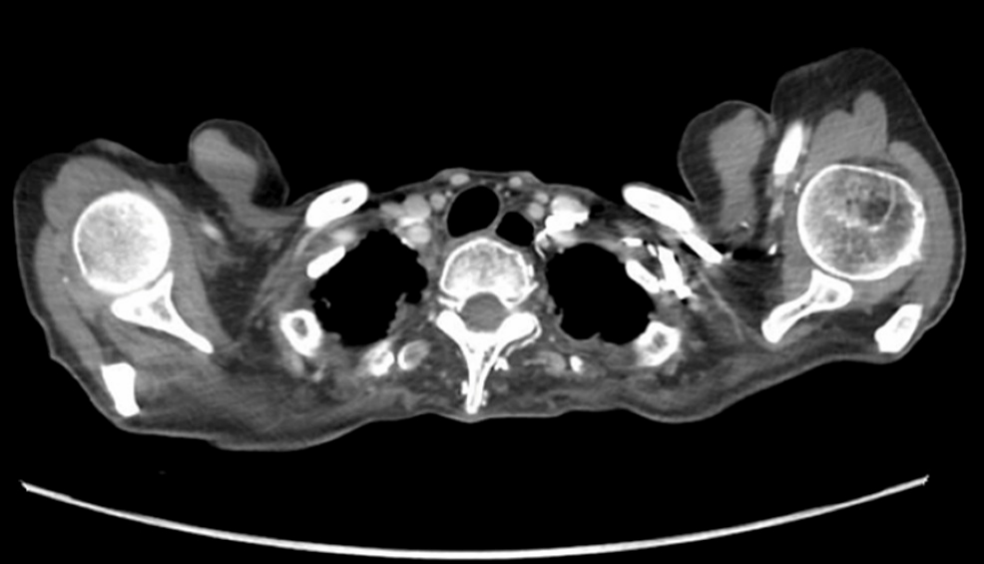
Head and neck CT scan with IV contrast Mucus plugging seen in the right lower lobe bronchus with small subpleural ground-glass opacities in the lower lobes bilaterally could be aspiration/infection.
